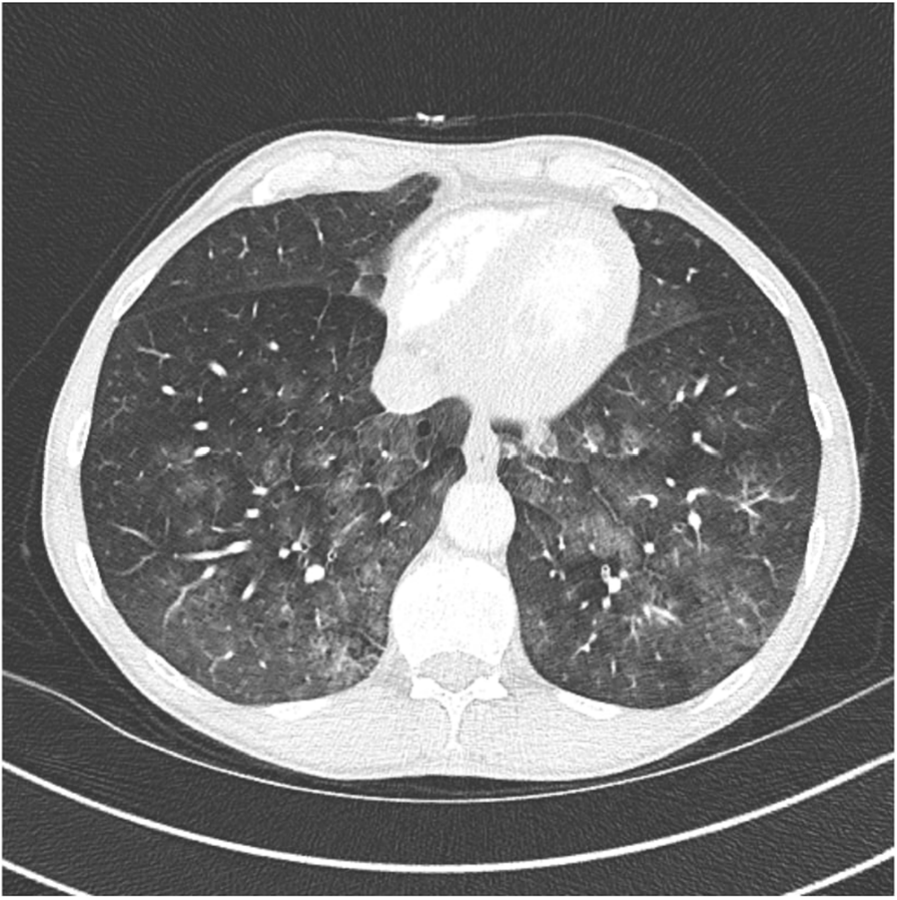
CT showing central ground glass opacities in the lower lobes, suggesting DAH
